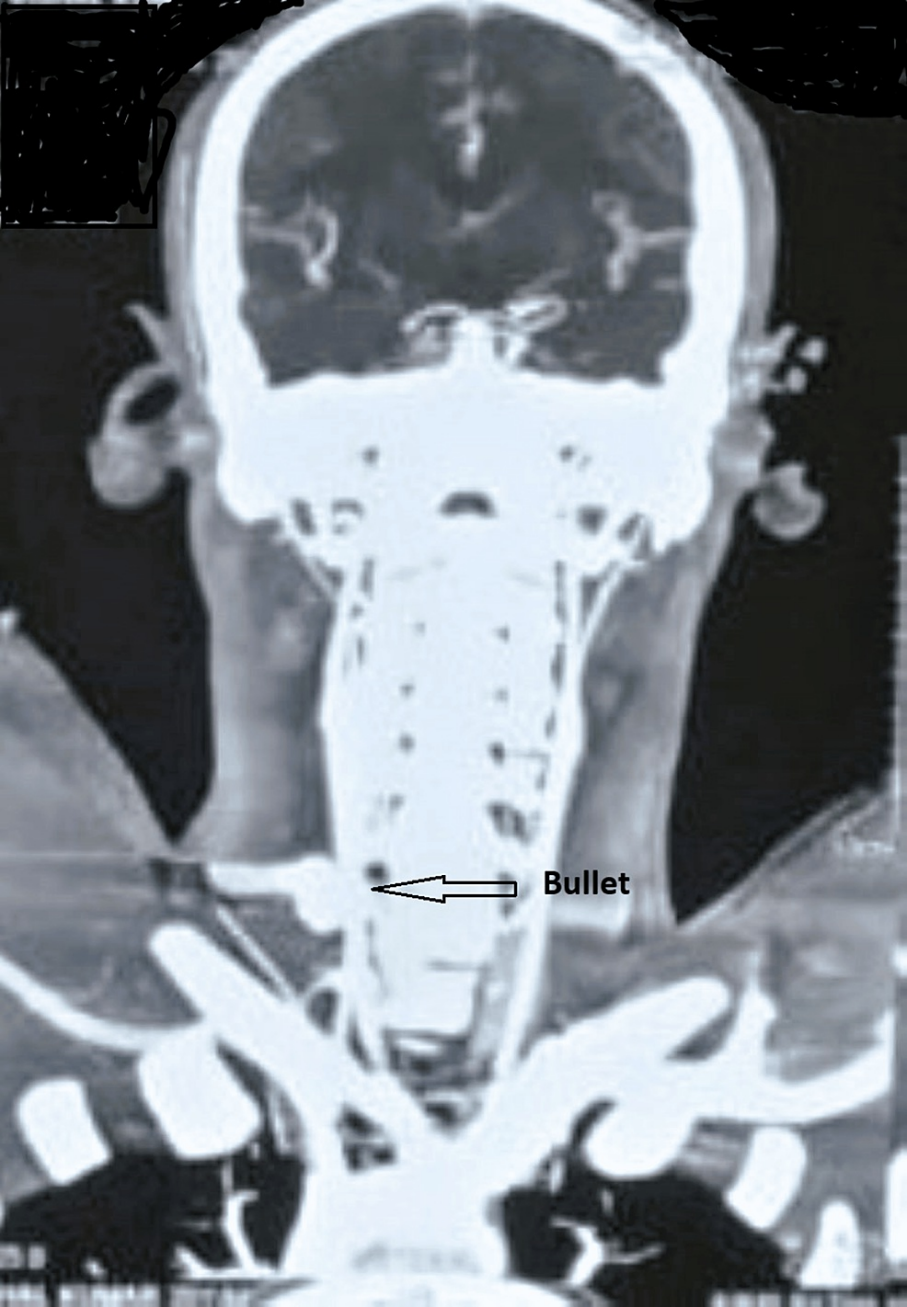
CT-angiography shows no contrast leak and a bullet marked with an arrow. CT: computed tomography.
